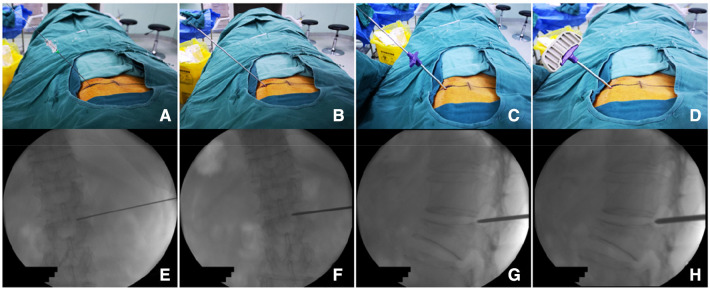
Procedure of percutaneous puncture to the bony entry point. (A) puncture needle puncture positioning to *P* point and local anesthetic injection; (B) puncture sleeve replacing the puncture needle; (C) working sleeve replacing the puncture sleeve; (D) after anchoring the bony entry point, replacing it with a Jamshidi needle to further enter into the vertebral body. (E–H) Represents the situation monitored by x-ray during operation.
